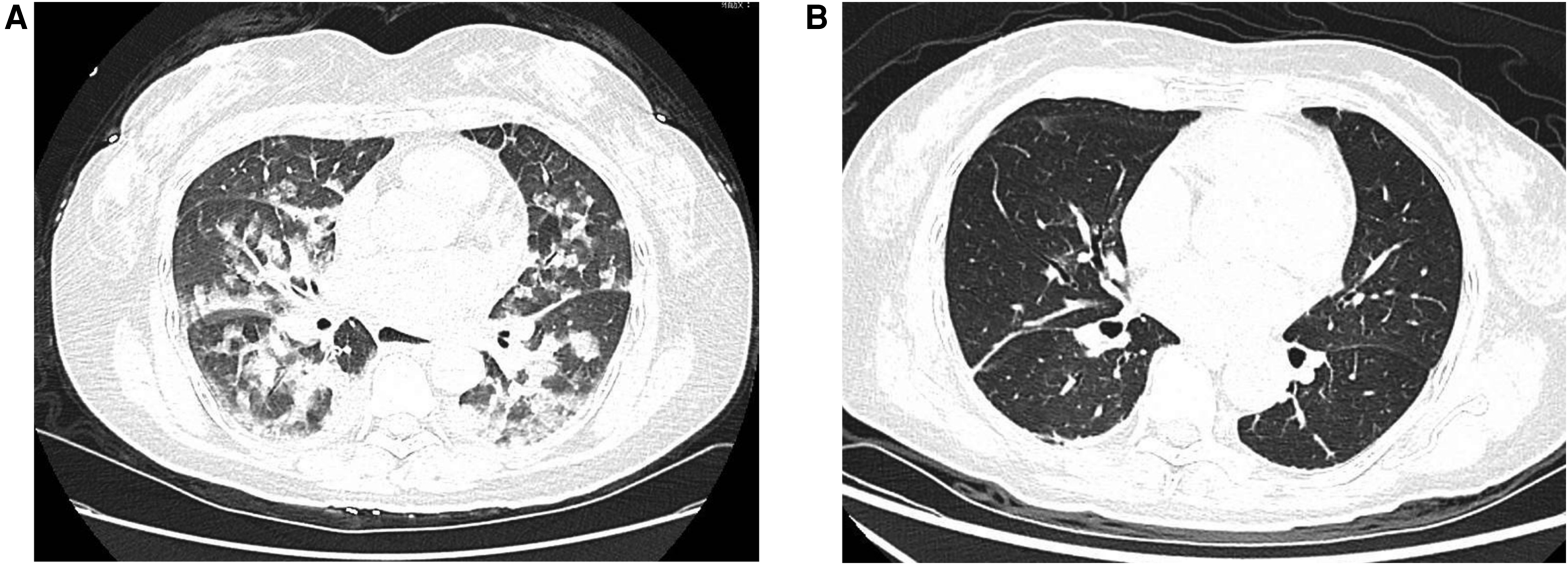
Chest computed tomography (CT) scans. (A) The initial chest CT scan performed upon admission demonstrated bilateral pulmonary edema. (B) A repeat chest CT scan at discharge showed complete resolution of the previously noted pulmonary edema.
